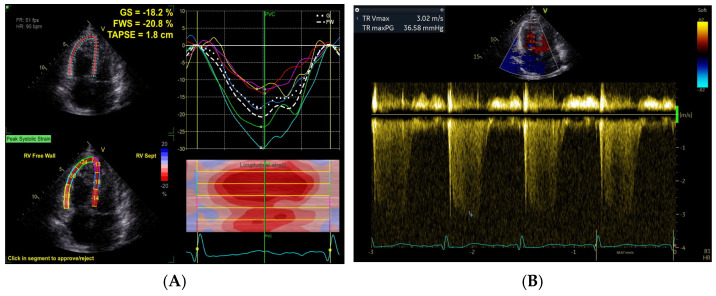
Example of echocardiographic measurement of RVFWLS and PASP: (A) Speckle tracking analysis for right ventricular free wall longitudinal strain (RVFWLS, −20.8% in this case), the different colored curves represented strain–time curves of different segments; (B) the TR pressure gradient was calculated from the continuous-wave Doppler TR velocity by the simplified Bernoulli equation. PASP was calculated by the formula PASP  =  4  ×  (peak velocity of TR)^2^  +  estimated right atrial pressure (PASP, 52 mmHg in this case). The RVFWLS/PASP ratio was manually calculated as the absolute value of RVFWLS divided by PASP, yielding 0.400%/mmHg in this case.
